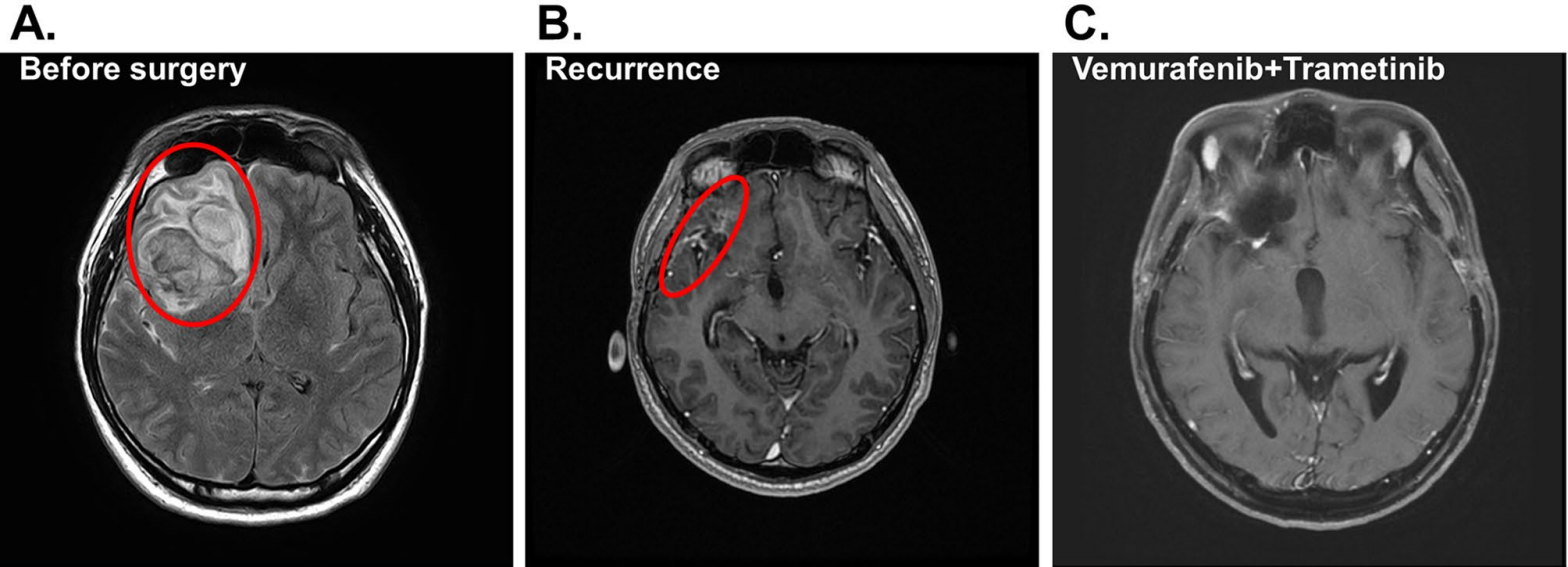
Radiological response of the brain lesion of patient #1 to BRAF inhibition. (A) Axial MRI before surgery with the tumor area circled. (B) The follow-up MRI image after surgery. (C) The follow-up axial image after combined treatment by vemurafenib and trametinib. Patient shows complete response.
